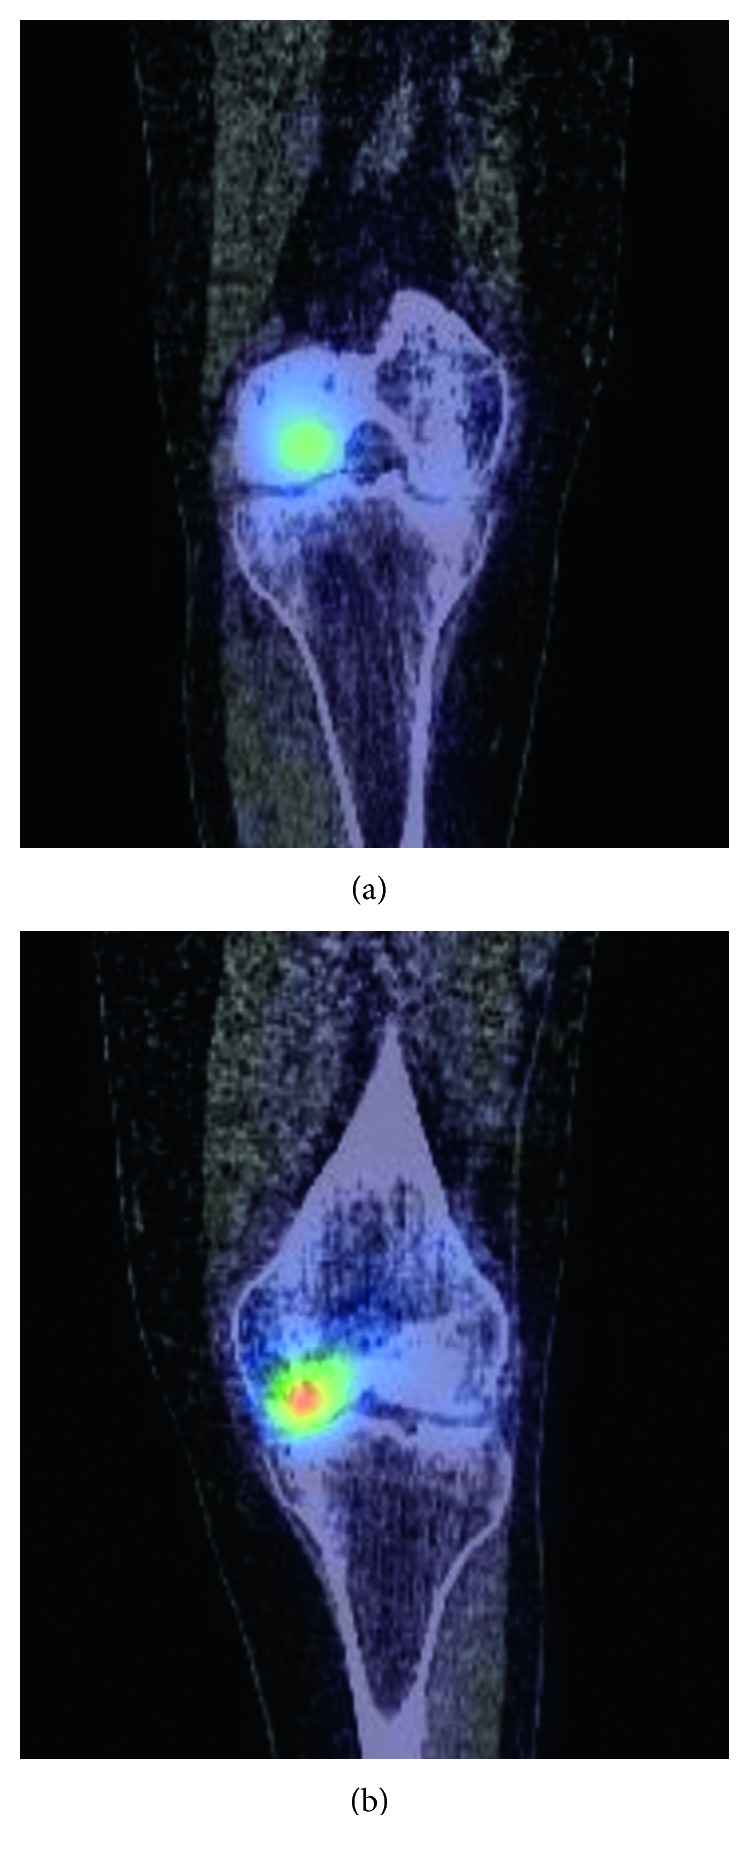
(a) and (b) Sagittal CT component of SPECT showing increased focal tracer uptake in the left medial femoral condyle.
